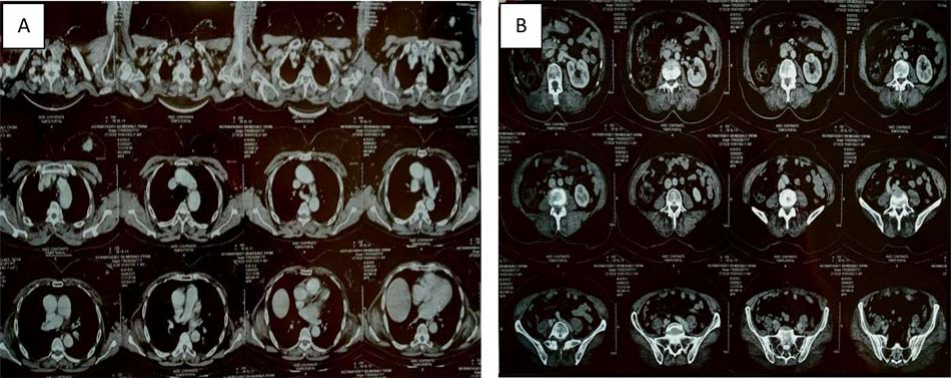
Thoracic-abdominal and pelvic computed tomography (CT) images showing on the thoracic level (A) a poorly limited mass measuring 27 × 22mm in the upper outer quadrant of the left breast (B) and a single left kidney at the abdomino-pelvic level.
